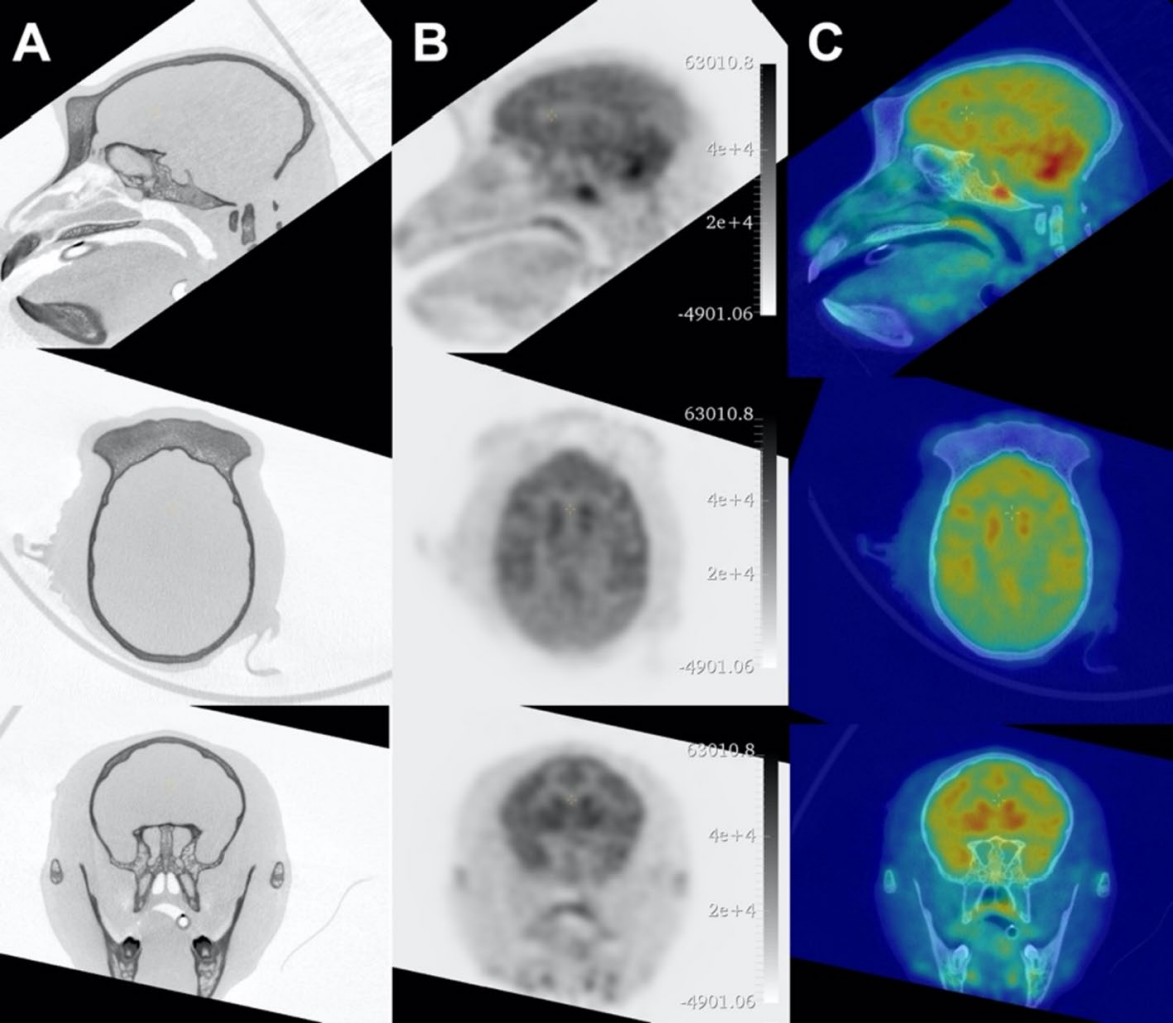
Coronal, sagittal, and axial slices of (column A) the CT, (column B) the PET, and (column C) the co-registered PET/CT images of the cerebral uptake of ^18^F-FDG of one Macaca fascicularis
